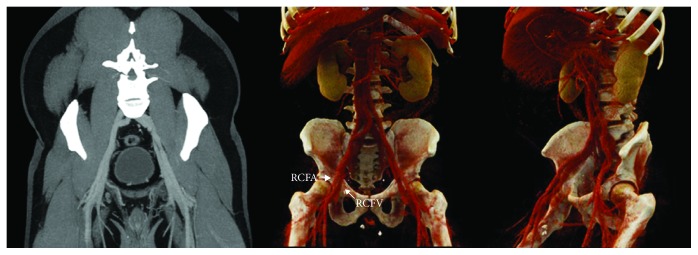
MIP (maximal intensity projection) and 3D cinematic rendering images of the CT venogram performed 1 month after the ultrasound exam were normal, with no evidence of residual venous thrombus or traumatic pseudoaneurysm.
